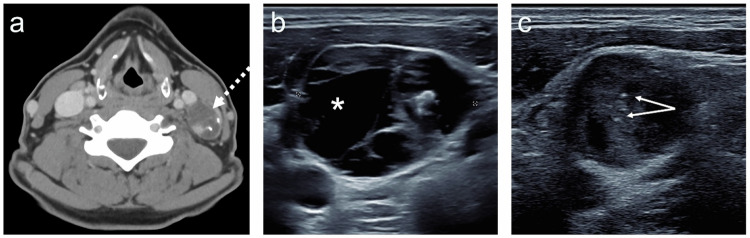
a Incidental CT finding of an abnormal left cervical (level 3) lymph node (dotted arrow) containing calcification and cystic spaces in a patient with small ITNs. b, c US demonstrated mixed isoechoic solid and anechoic cystic consistency (asterisk) with punctate echogenic foci consistent with microcalcification (arrows). FNA from the level 3 lymph node demonstrated papillary thyroid carcinoma with surgical confirmation (pT1 8 mm micro papillary thyroid carcinoma pN1b)
